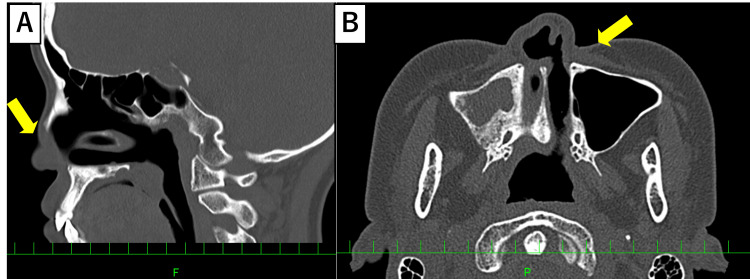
CT findings in Case 1, showing left alar and septal defect with cartilage thinning (yellow arrow) (A) Sagittal view. (B) Axial view
